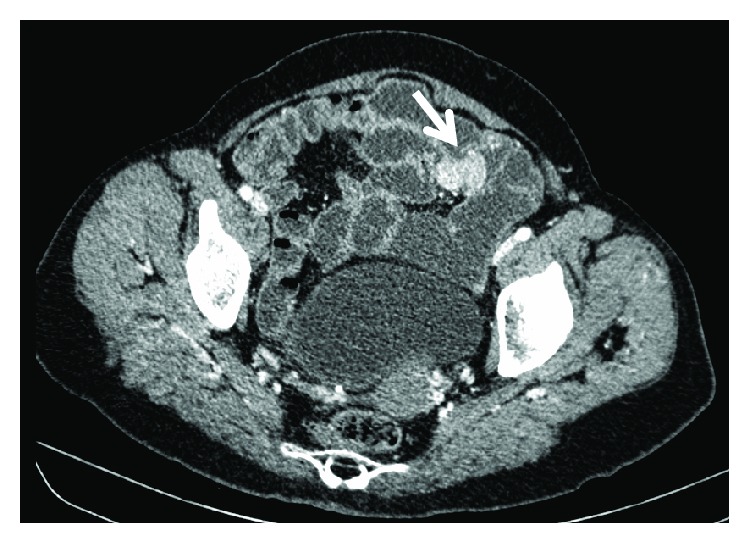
CT enterography—axial CT image with 20-24 mm jejunoileal lesion compatible with GIST.
